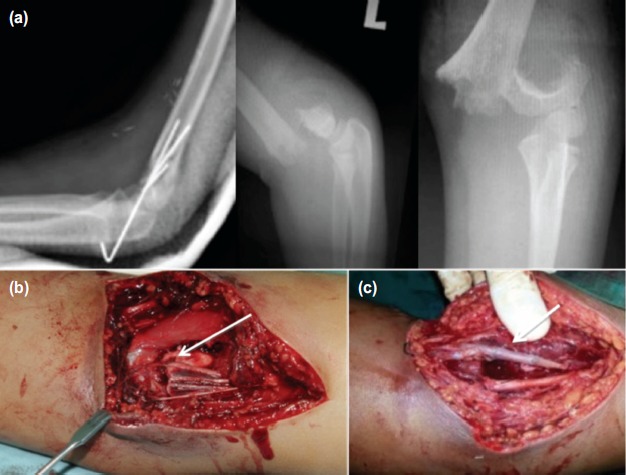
An 11 year-old boy with a displaced supracondylar fracture and absent distal pulses; (a) CT angiography revealed a 3cm segment loss of the brachial artery with good collateral. (b) The artery was trapped and constricted by the proximal fracture fragments. (c) Bypass with reverse saphenous graft was able to establish the flow.
